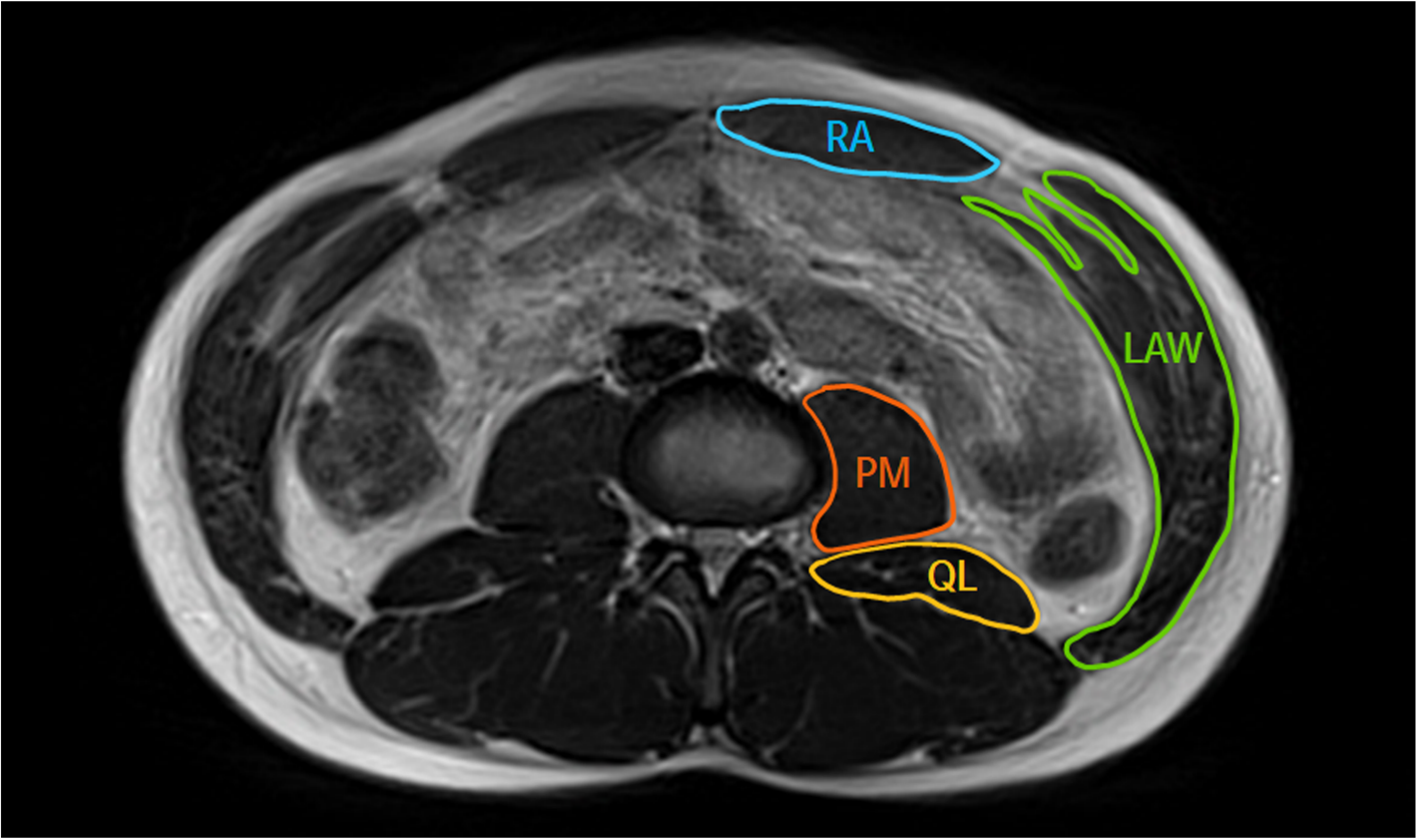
Magnetic resonance imaging of abdominal muscles and paraspinal muscles at L3–4 level. RA = Rectus abdominis; LAW = Lateral abdominal wall; QL = Quadratus lumborum; PM = Psoas major
